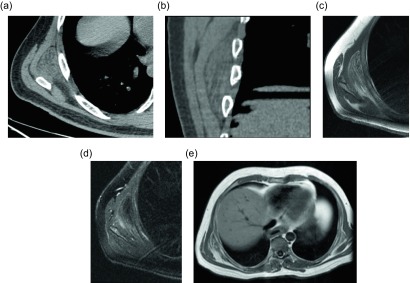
Elastofibroma dorsi: 44-year-old male with a painless mass in the right posteroinferior scapular region. Axial (a) and coronal (b) non-contrast CT demonstrate a poorly-circumscribed mass, deep to the serratus anterior muscle, with tissue of attenuation similar to muscle and internal strands of fat. Subsequent axial T1W (c) and T2W fat saturated (d) images have a similar appearance with signal intensity comparable to muscle and internal bands of fat which suppress on fat saturated images. Larger field of view T1W (e) image from the same study demonstrate a small mass with similar signal intensity within the left subscapular region.
